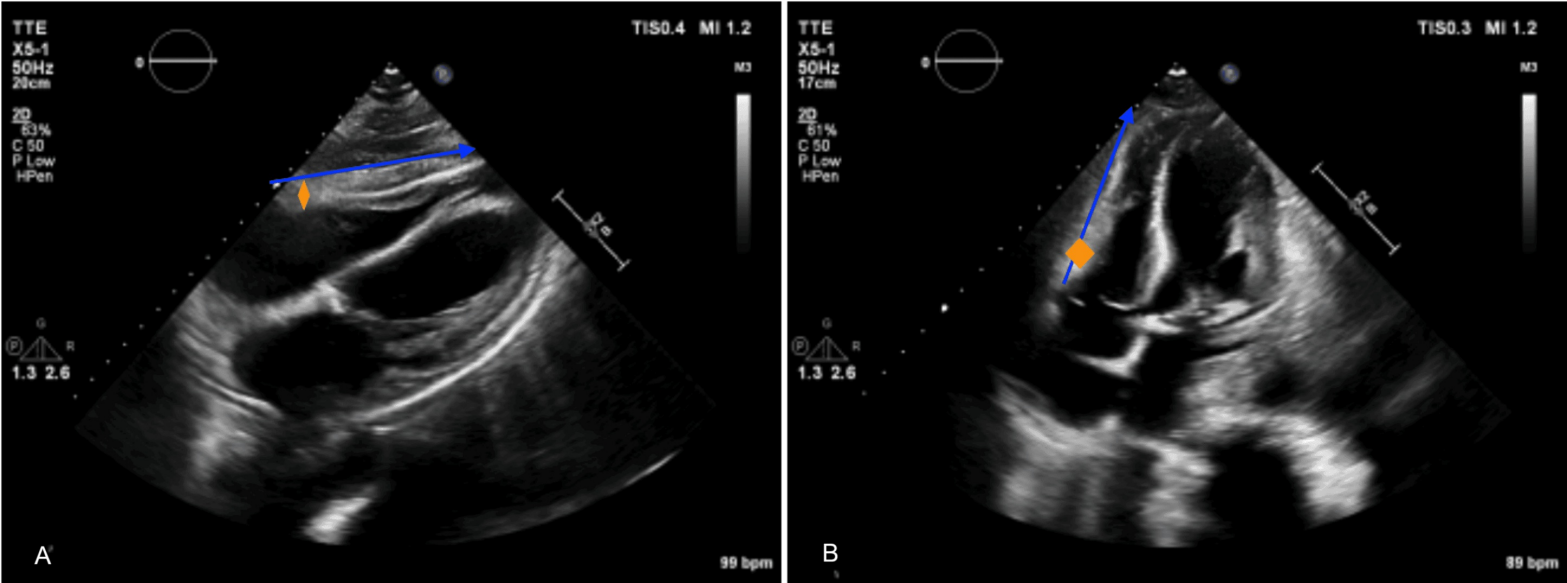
TTE after several weeks of corticosteroid therapy, still undergoing tapering regimen (A: subcostal view; B: Four-cavity view) Transthoracic echocardiography (TTE) shows regression of the pericardial mass, with a reduction in the compressive effect on the right ventricle and decreased pericardial thickening. Blue line = length 5.1 cm; orange line = width 0.7 cm (compared with 7 cm × 1.5 cm previously).
